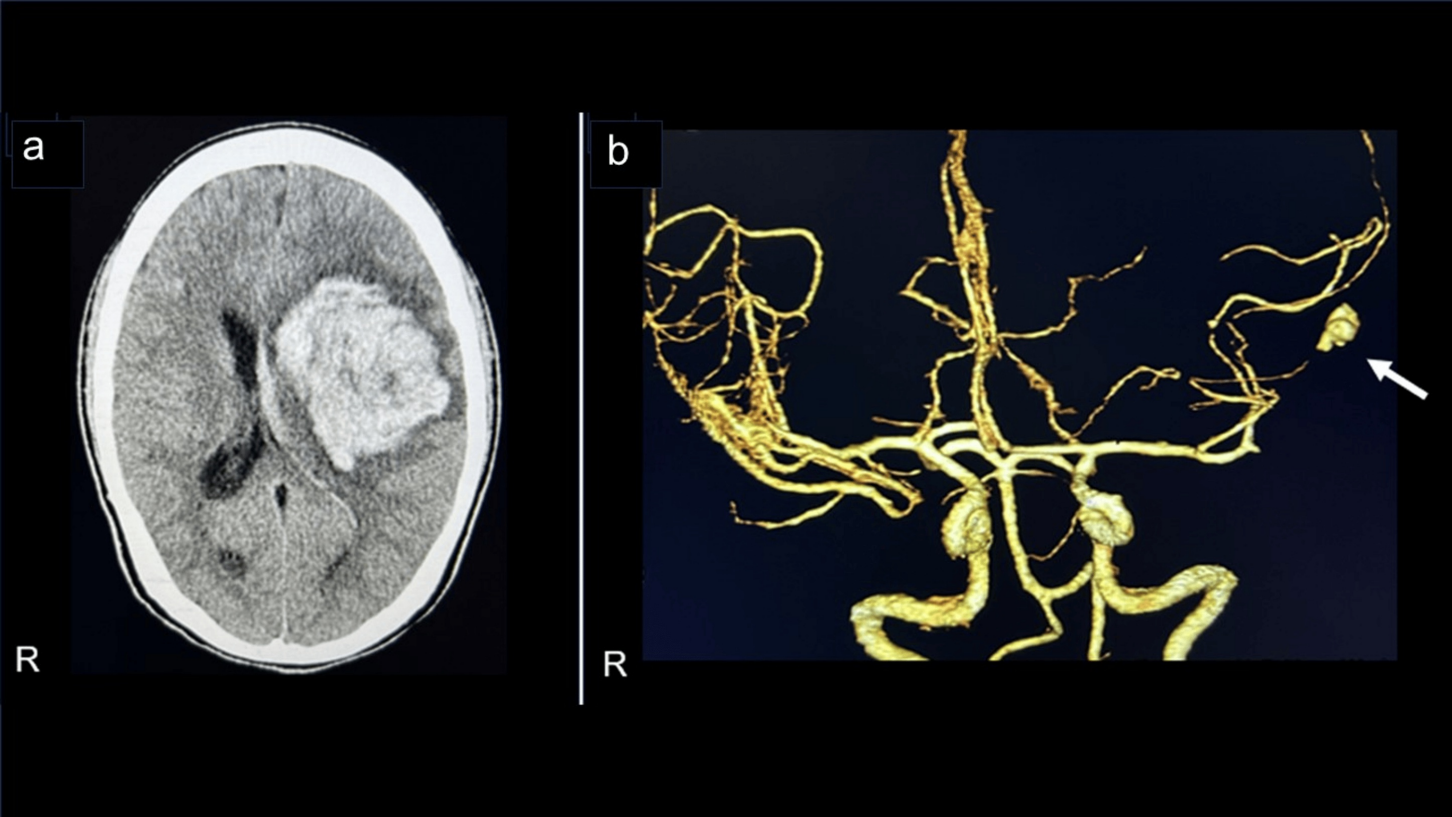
(a) Brain CT, showing intracranial hemorrhage in the left frontal lobe. (b) Cerebral angiography CT, showing aneurysm in the distal left middle cerebral artery (arrow) CT: computed tomography
